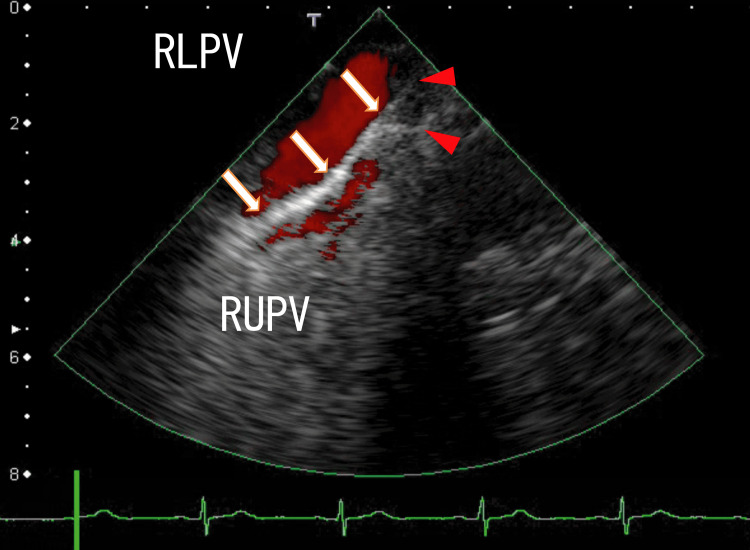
TEE images showed that the LA thrombi extended from the right upper pulmonary vein (RUPV) and reached the anterior wall of the LA. The arrows indicate white LA thrombi, which are connected around the exit of the RUPV and the anterior wall of the LA. The arrowheads indicate the attachment regions of the LA wall, which are near the RLPV thrombus attachment regions. RLPV, right lower pulmonary vein; LA, left atrium; TEE, transesophageal echocardiography
